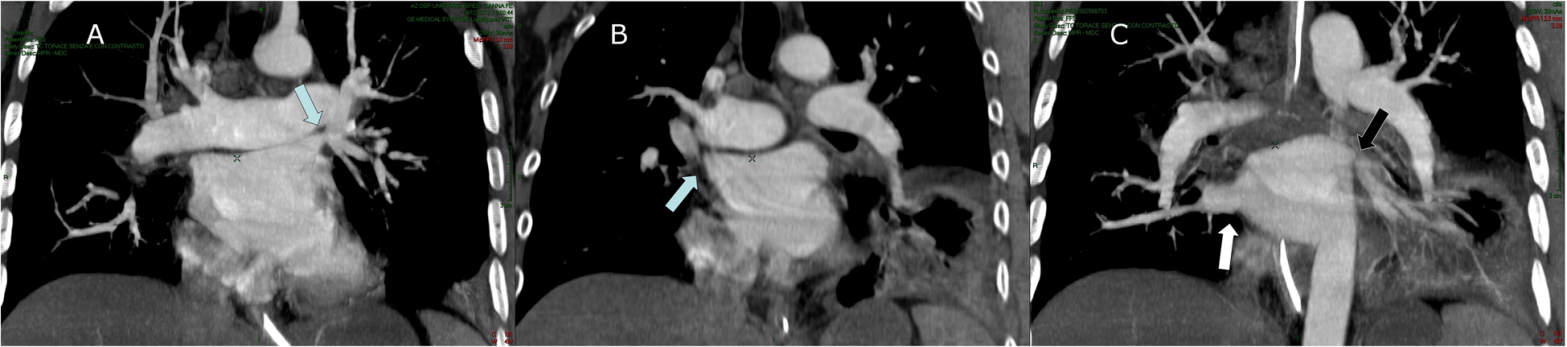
a Computed tomography angiography A-P projection showed the concentric severe stenosis of the left superior pulmonary vein (arrow). b CT angiography A-P projection showed 50 % stenosis of both right superior pulmonary (arrow). c CT angiography A-P projection showed ectatic right inferior vein (white arrow) and stenosis of left inferior pulmonary vein (black arrow)
